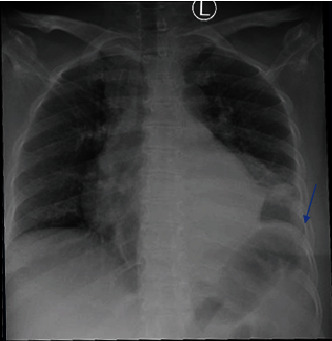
Chest x-ray done with patient presentation to the emergency department showing left lower lobe opacification and obliteration of the costophrenic angle due to pneumonia complicated by parapneumonic effusion (Blue arrow).
